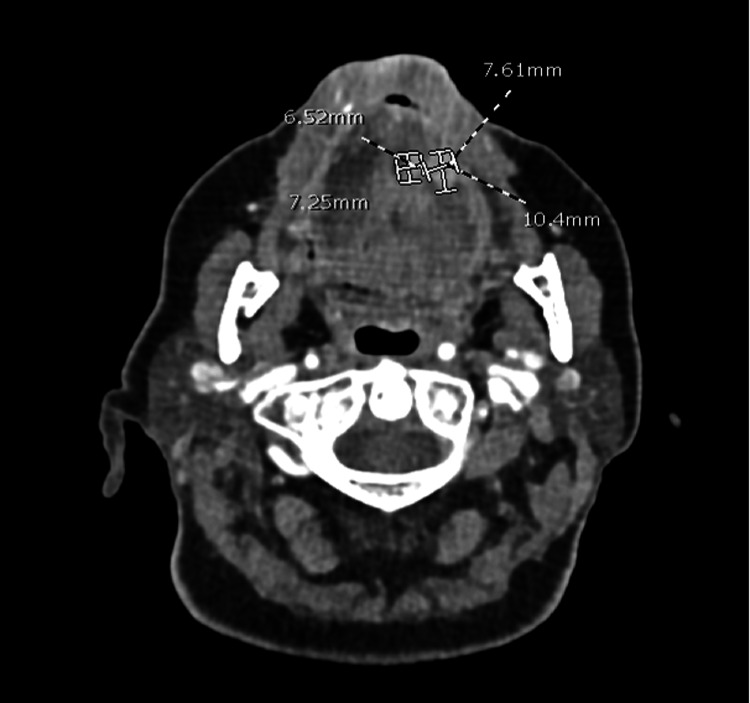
CT of the face, axial view The image shows postcontrast heterogeneous enhancement in the left aspect of the genioglossus muscle, as well as two nodular images with slight homogeneous postcontrast enhancement of 7.2 x 6.5 mm and 10.4 x 7.6 mm in their anteroposterior and transverse diameters, suggesting post-surgical tumor activity. CT: Contrasted computed tomography
